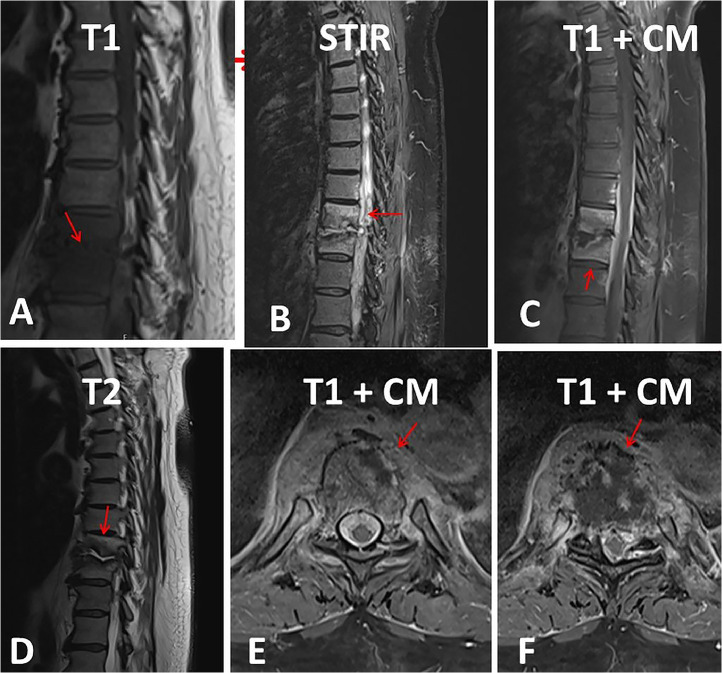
MRI of the spine with pronounced spondylodiscitis. The Th9/10 T2-weighted disc shows high signal intensity and is significantly narrowed (panels B and C). The base plate of the BWK 9 and the cover plate of the Th10 are irregular and show pronounced signal changes; There is a reduction in the height of both vertebral bodies, with the depression in the base plate of Th9 being emphasized on the right and the depression in the cover plate of BWK 10 being emphasized on the left (panels A, B, C, D). In the STIR sequence there are hyperintense bone marrow signal changes, which are shown with a low signal on T1 (panel B). After contrast, significant enhancement of Th9 and Th10 and the surrounding soft tissues (panels C, E, F). At the Th9/10 level, fusiform contrast enhancement dorsal to the vertebral bodies in the spinal canal over a longitudinal extent of 7.8 cm, with a width of up to 6 mm, corresponding to an epidural abscess, with consecutive constriction of the dural sac (panel B). The myelon itself shows no signal change.
